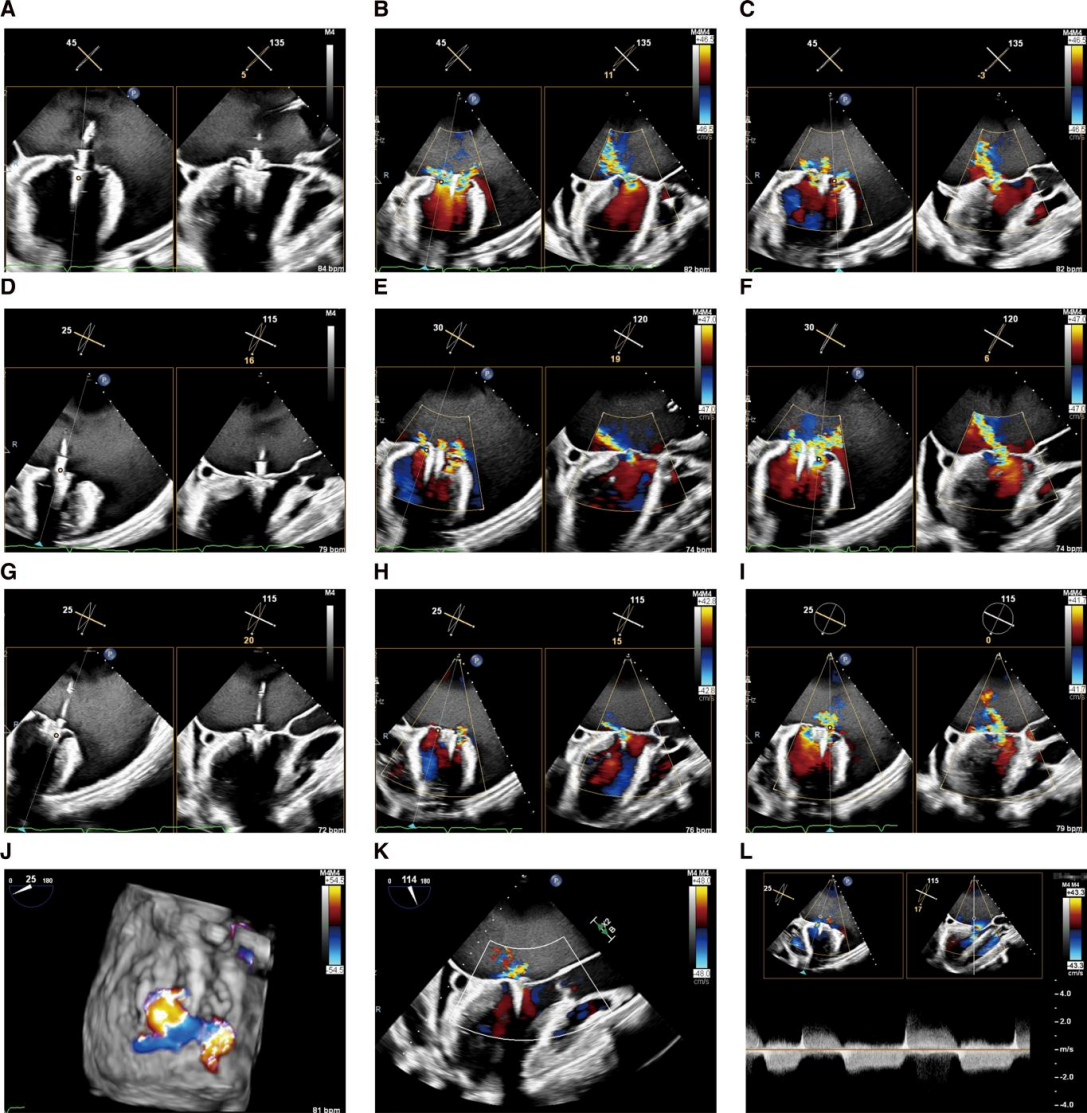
Intraprocedural transesophageal echocardiogram. (*A–C*) Residual medial and lateral regurgitation following deployment of the first MitraClip XTW device. (*D–F*) Reduction in regurgitation after implantation of a second MitraClip XT device medial to the first clip. (*G–I*) Further reduction after deployment of a third MitraClip XT device lateral to the first clip. (*J–L*) Final result showing mild residual mitral regurgitation and a mean transmitral gradient of 5 mmHg.
